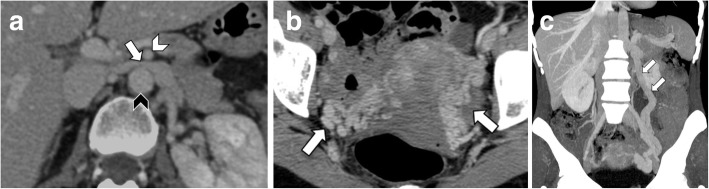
A 50-year-old woman with anterior nutcracker syndrome. a Axial contrast-enhanced CT image on the portal venous phase shows the typical “beak sign” (arrow) with left renal vein compression between the aorta (black arrowhead) and superior mesenteric artery (white arrowhead). b CT image at the level of the pelvis demonstrates multiple pelvic varices (arrows). c Coronal reformatted CT MIP image shows marked dilatation of the left ovarian vein (arrows)
